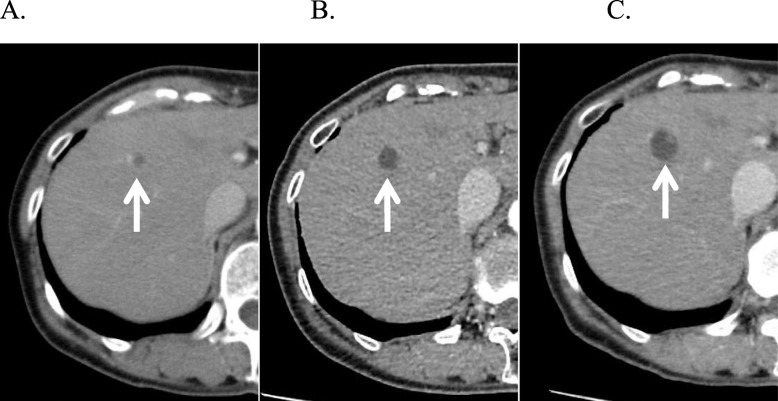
Computed tomography findings. Computed tomography scans showed a a 7-mm cystic nodule in 2012 (white arrow), b an 11-mm cystic nodule in 2016 (white arrow), and c a 17-mm cystic nodule in 2017 (white arrow) as well as a mural nodule in the wall
